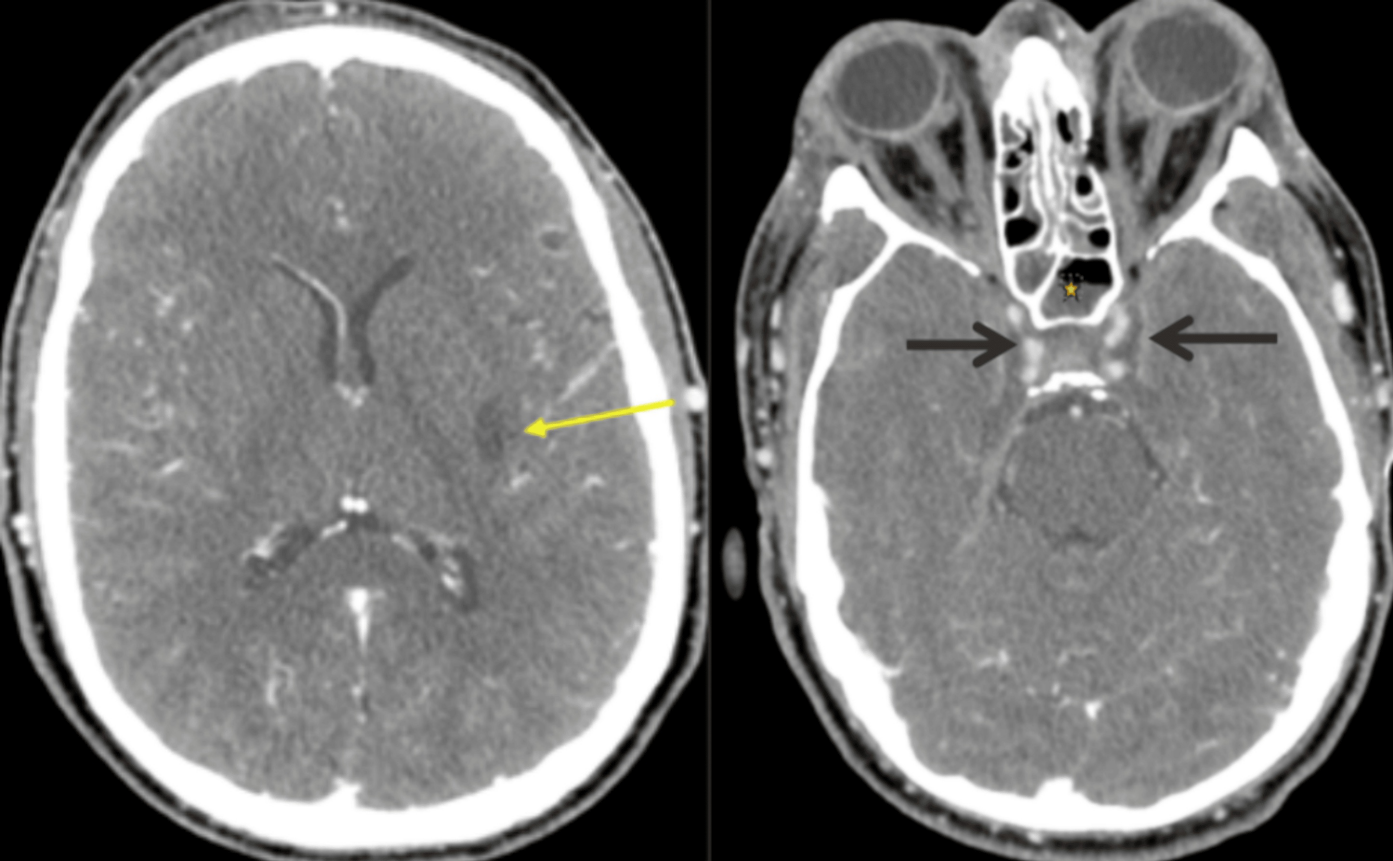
CT venogram of the head (day five) left: infarct in the left lentiform nucleus; right: filling defect in bilateral cavernous sinuses (arrows) consistent with thrombosis, and air-fluid levels in the sphenoid sinuses (star)
